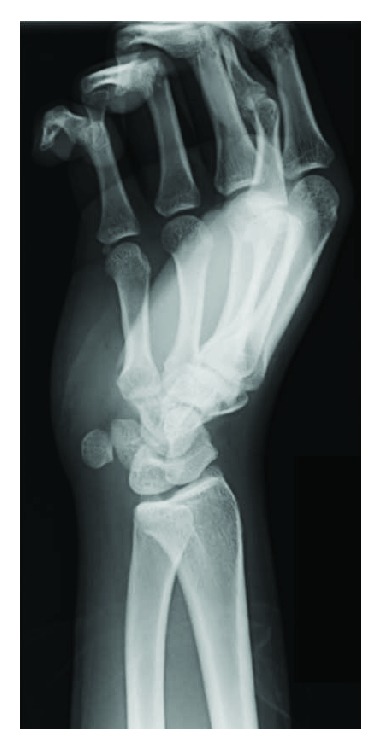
Postoperative radiograph in supinated oblique position.
